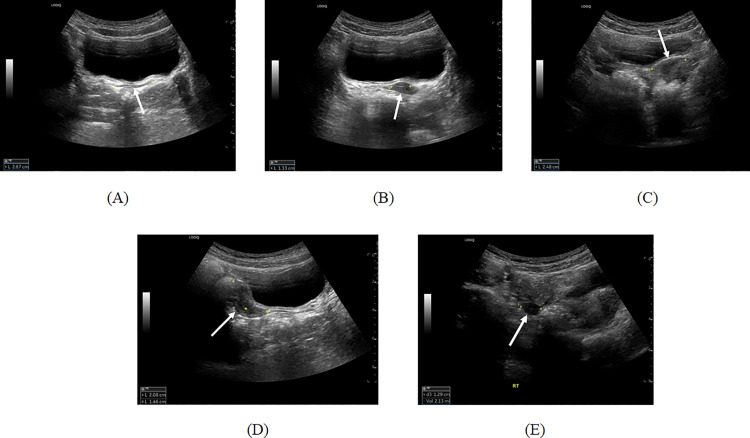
Pelvic US example of an 8-year-old girl with P-CP. US demonstrated (a) a vaginal width of 2.7 cm, (b) a cervix width of 1.3 cm, (c) a fundus width of 2.5 cm, (d) a uterine length of 3.5 cm, and (e) a right ovarian volume of 2.1 cc (arrows). The score for Model C was 19.4 (cutoff value > 16 for diagnosing P-CP) with a basal LH of 4.2 IU/L and estradiol of 60.8 pg/mL. US, ultrasound; P-CP, progressive central precocious puberty; LH, luteinizing hormone.
